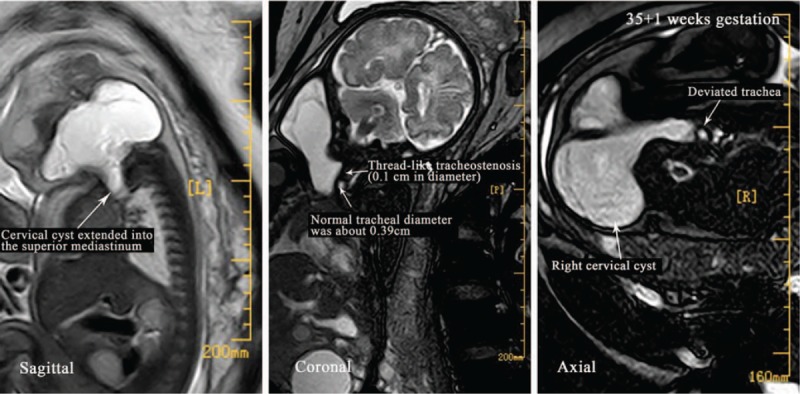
Fetal sagittal, coronal, axial MRI images at 35^+1^ weeks’ gestation (WG). MRI showed cyst growth to 3.3 × 8.2 × 7.5 cm and extended from the right neck into the superior mediastinum. The pharynx, trachea, and vessels were compressed and significantly deviated to the left. The inside diameter of the narrowest section of tracheostenosis appeared thread-like and measured only 0.1 cm.
